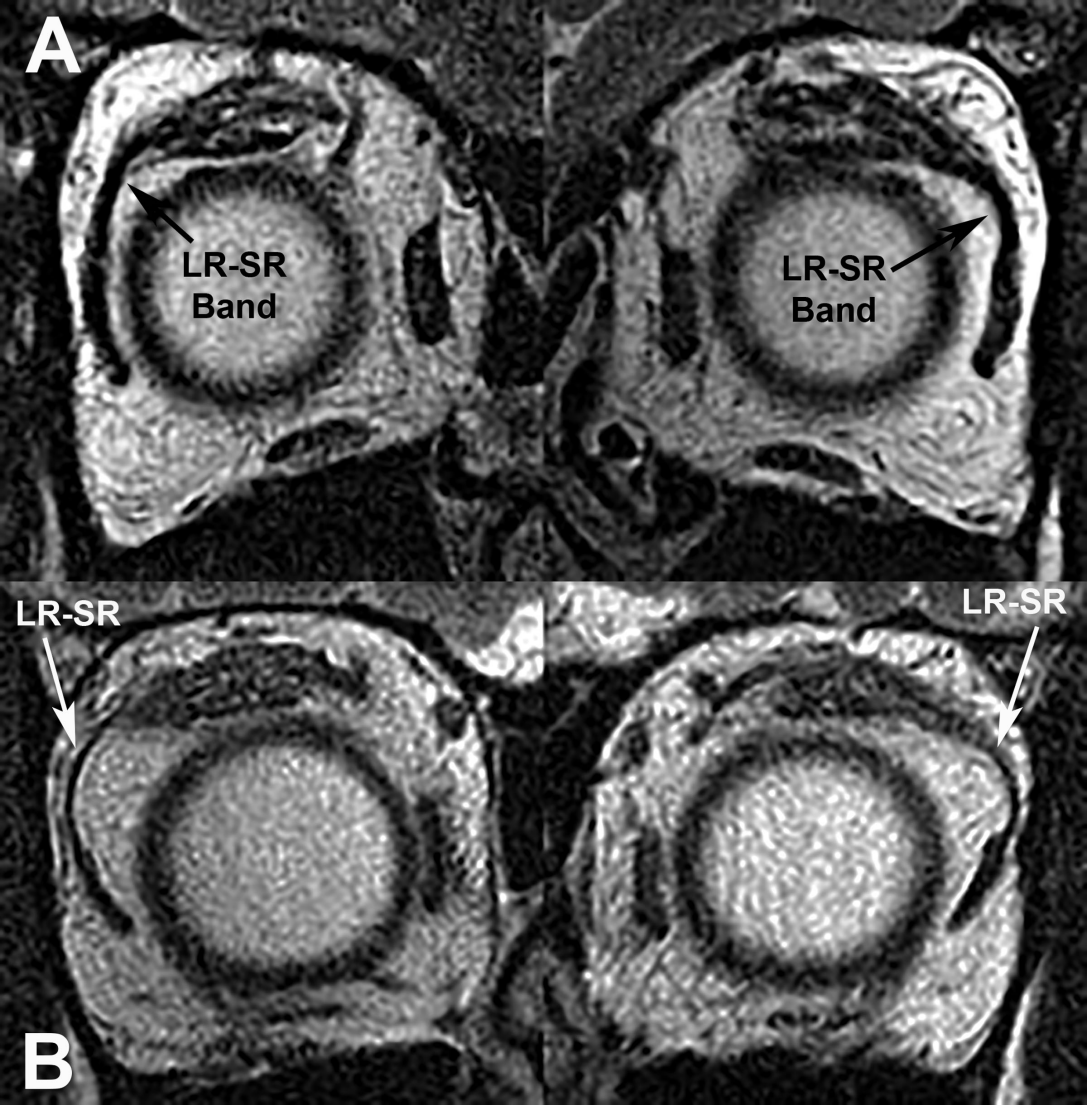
Quasi-coronal MRI in anterior planes in the subjects from Figure 1 with right hypertropia due to (A) SOP, demonstrating bilaterally robust LR–SR band ligaments and LR muscles in similar vertical position to the MR muscles, and (B) SES with attenuated, bulging LR–SR bands and inferiorly displaced, obliquely oriented LR muscles.
